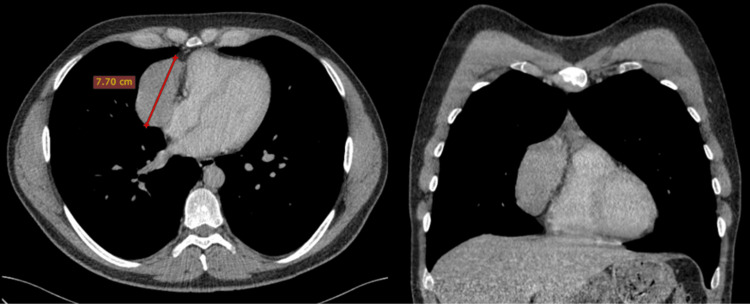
Axial and coronal contrast-enhanced CT CT: Computed tomography Axial and coronal contrast-enhanced CT: Well-defined hypovascular homogeneously enhancing anterior mediastinum mass along the right border of the heart.
